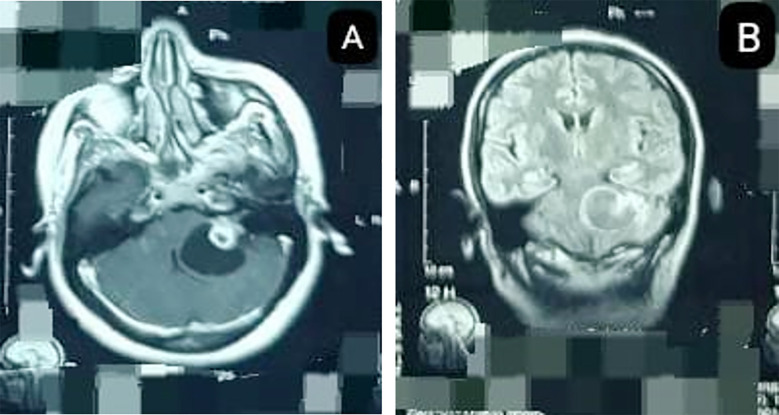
Contrast-enhanced MRI (CE-MRI) brain (A) axial & (B) coronal view showing heterogeneous left CP Angle SOL.
